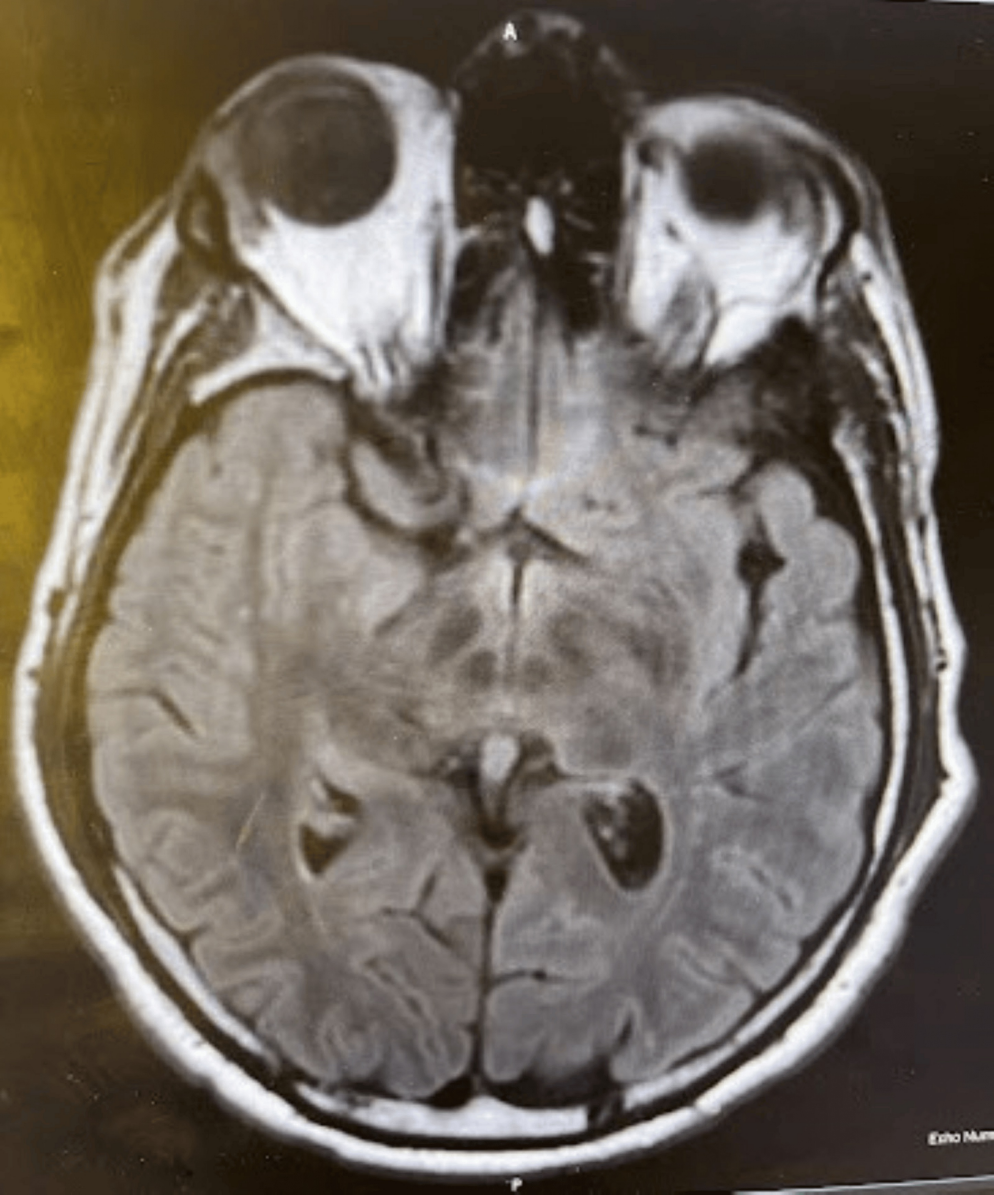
Magnetic resonance image (MRI) of the brain with and without contrast non-lesional for seizures.
